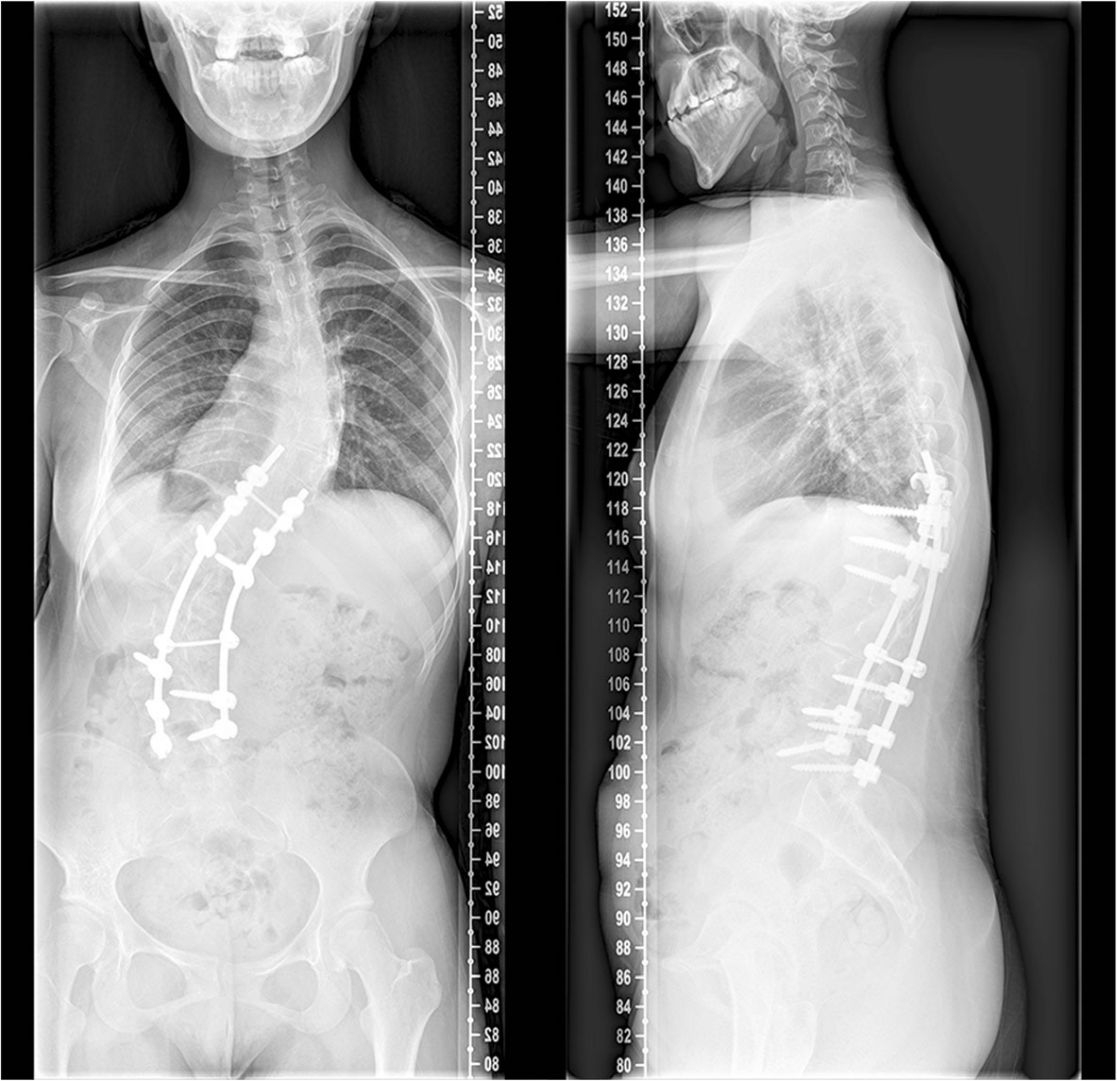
Standing anteroposterior and lateral radiographs of 8 months after operation
